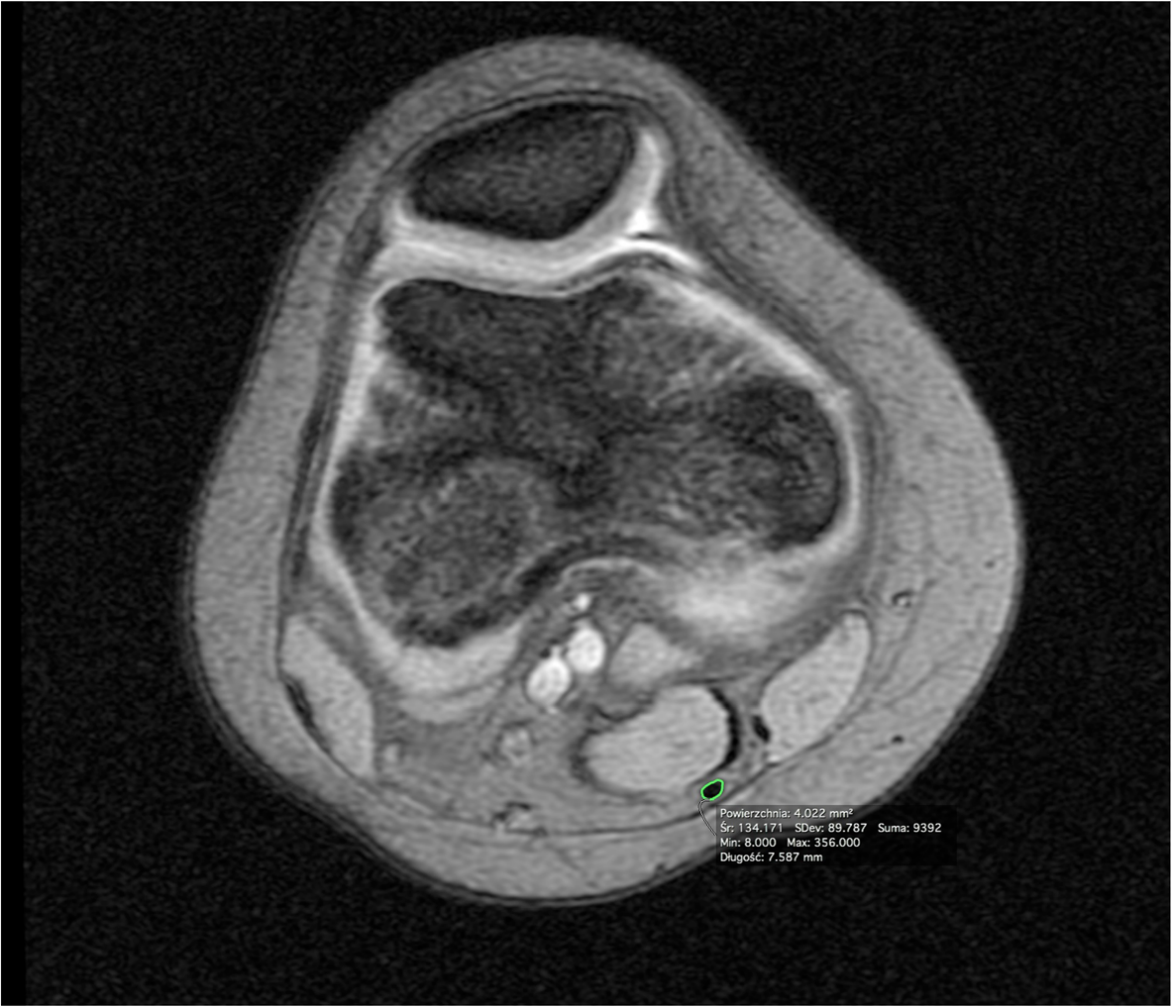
Knee MRI at the femoral growth plate level (axial view). A cross-section area of the ST tendon
